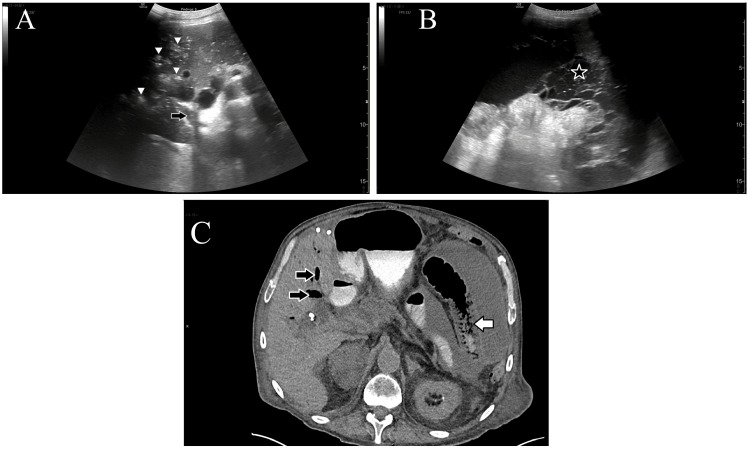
Case 2 - POCUS images and CT A: B-mode image of the liver shows collections of hyperechoic gas (white arrowheads) within the parenchyma, as well as gas (black arrow) flowing within the portal vein. B: A loculated fluid collection (star) is visible in the upper abdomen. C: CT image demonstrates dilated small bowel (white arrow) with mucosal sloughing and gas (black arrows) within the portal veins. B-mode: brightness mode; POCUS: point-of-care ultrasound; CT: computed tomography
